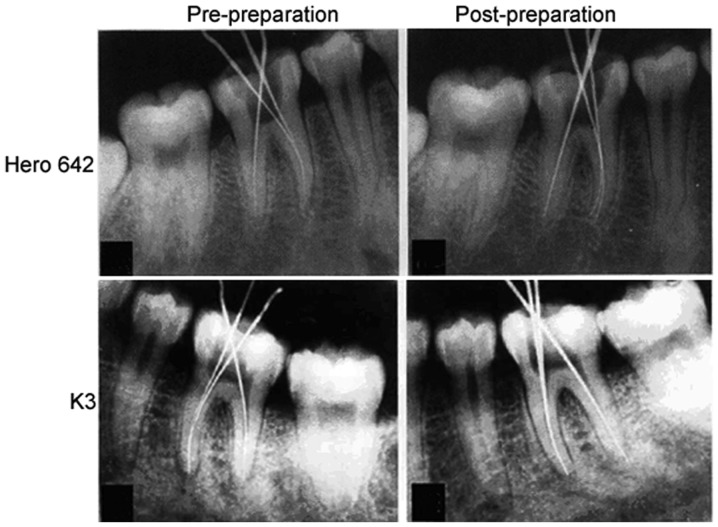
Representative radiographs of the patients treated with Hero 642 and K3 instruments.
